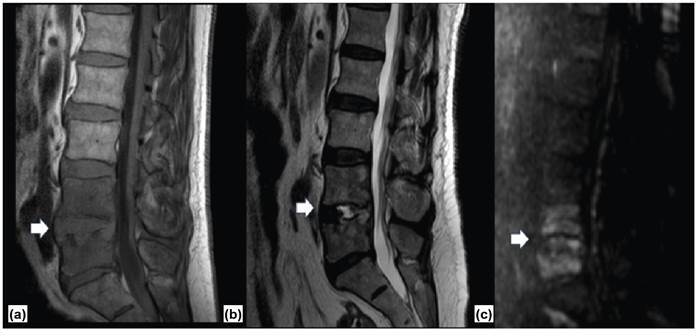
Sagittal images of spine MRI showing an infectious process in the intervertebral disc and vertebral bodies at L4-L5, characterized by hypointensity on T1 weighted images (WI) (A), hyperintensity on T2 WI (B) and restricted diffusion on diffusion WI (C). Gadolinium contrast medium injection was not indicated because the patient had a chronic dialytic kidney disease.
